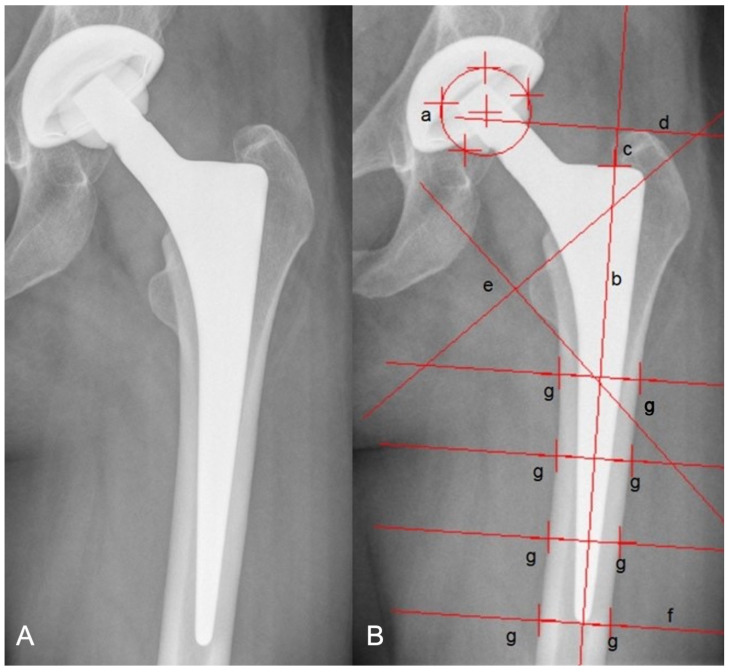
Anterior to posterior X-ray shows a CBC stem (A) and one with EBRA-FCA references (B): (a) head points, (b) stem axis, (c) stem shoulder, (d) major trochanter line, (e) minor trochanter lines, (f) tip-of-stem line, (g) points at femoral bone contour.
